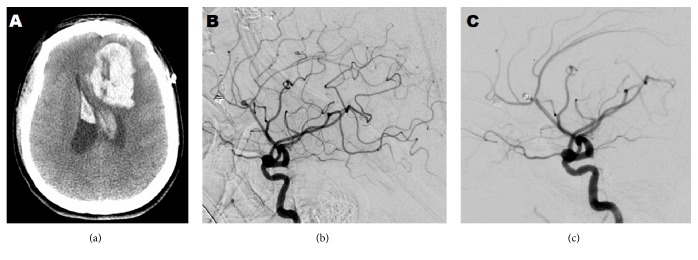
(a) 5 days after discharge in 2016: CT head shows large intracerebral hematoma. (b) A diagnostic cerebral angiogram less than 3 weeks from previous imaging demonstrating interval development of a saccular aneurysm at the callosomarginal and pericallosal bifurcation. (c) Cerebral angiogram postcoiling.
